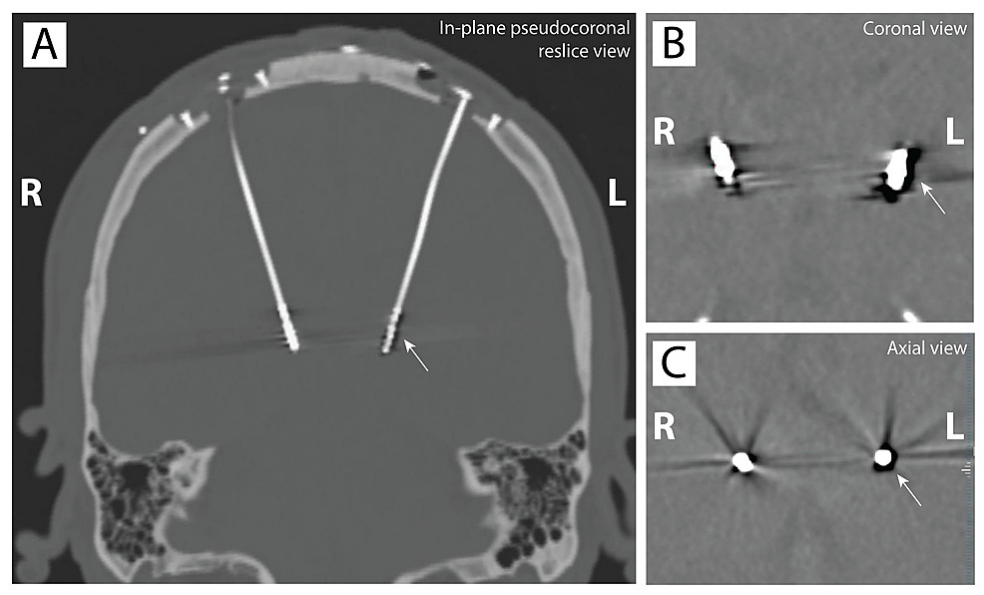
Perioperative CT image from example case 1 (A, B) Coronal (resliced along the plane of the left lead) and (C) axial (resliced orthogonal to the plane of the left lead) non-contrast head CT after ventral intermediate nucleus deep brain stimulation implantation showing air bubble surrounding the left electrode in example case 1.
